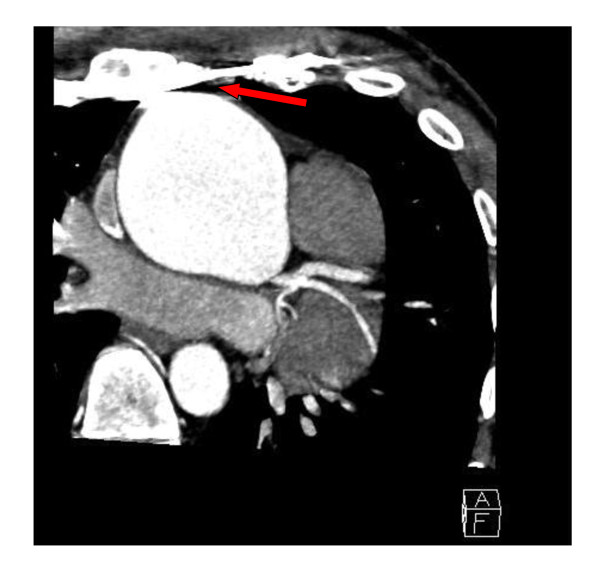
Follow-up CT-scan. Red arrow demonstrates the close relationship between one of the metal bars and the annuloaortic ectasia.
